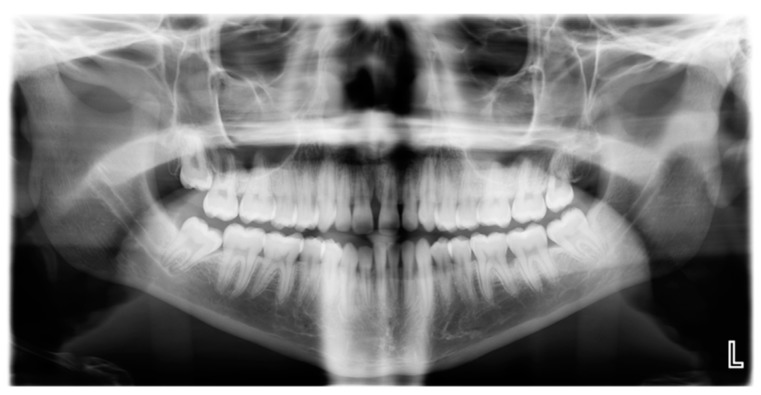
Radiograph of subject with Localized Aggressive Periodontitis: Panoramic radiograph showing extreme bone loss in first molar on left side in a 19-year-old patient.
